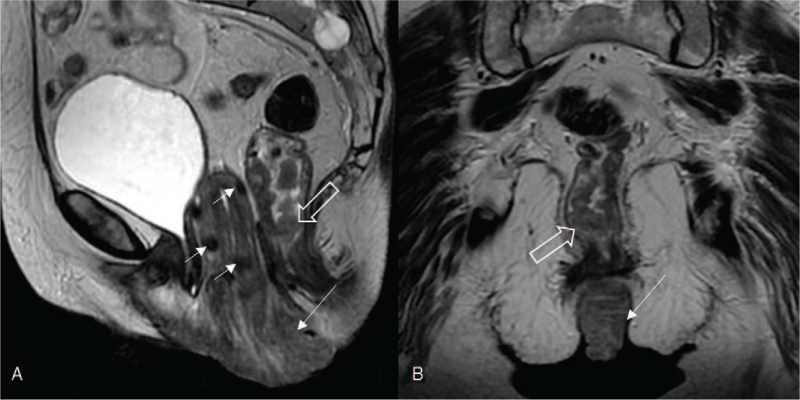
Sagittal (A) and coronal (B) T2-weighted magnetic resonance images. The prolapsed cervix of irregular morphology with the disrupted cervical stroma ring (long arrow). The thickened distal part of the rectal and anal canal wall (hollow arrow). Multiple round low signal myometrial lesions – uterine fibroids (short arrows).
